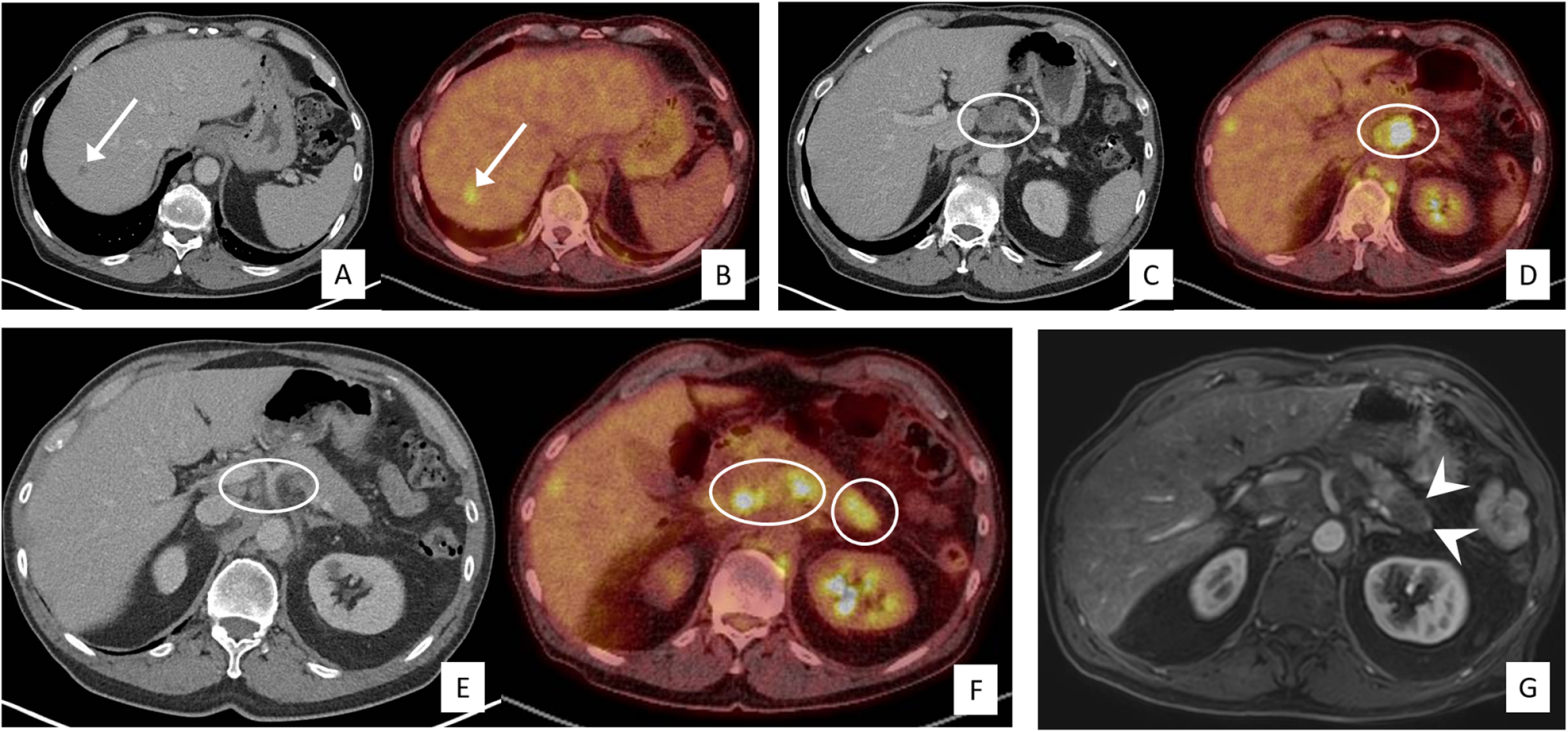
Patient (#17, see Supplement 1) diagnosed on contrast-enhanced CT and FDG-PET/CT with malignant liver lesions (arrows in A, B) and multiple pathologic upper abdominal lymph nodes (circles in C–F). On MRI, one of the lesions diagnosed on FDG-PET/CT as a peripancreatic lymph node was recognised to, in fact, concern a tumour in the pancreatic tail (arrowheads in G; arterial contrast-enhanced T1-weighted sequence shown). This tumour was not recognised as such on CT (C). This led to the correct final MRI diagnosis of a pancreatic adenocarcinoma as the underlying primary tumour
